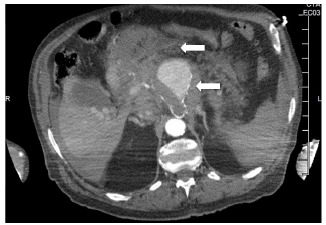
Computed tomographic angiography of the abdomen in axial view demonstrating active extravasation (top arrow) of the celiac artery aneurysm (bottom arrow).
